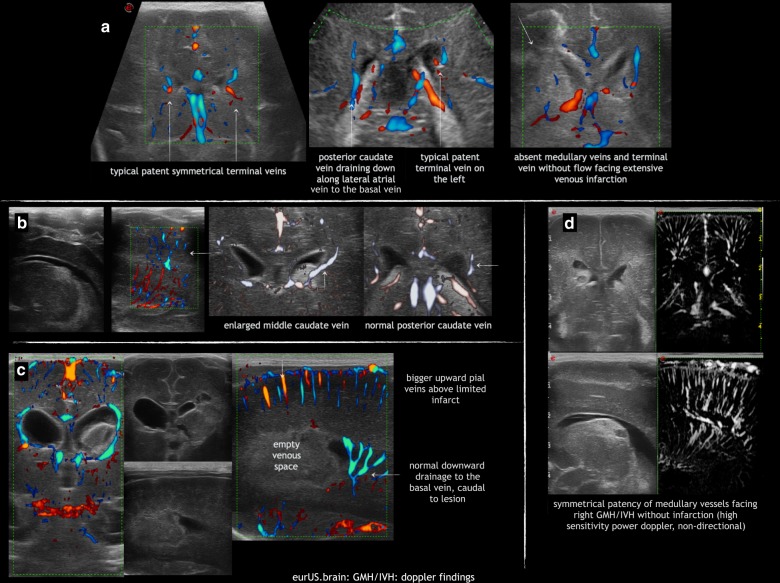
GMH/IVH: Doppler findings. a Asymmetry in terminal vein size, inversely related to size of ipsilateral direct lateral vein or lateral atrial vein; to be compared with absent terminal vein facing extensive venous infarction. b Apparent enlargement (congestion) of a vein by GMH. c Escape of blood from deep venous infarct along enlarged pial veins, above a Doppler-empty venous space. d Normal deep venous anatomy in GMH without infarction.
